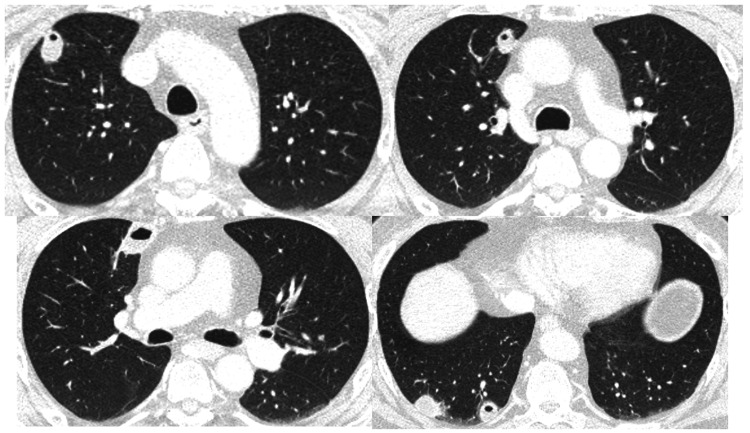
Chest CT on day two Multiple small abscesses were seen mainly in the patient's right lung. During the clinical course, a small amount of pleural effusion emerged in the right thoracic cavity.
